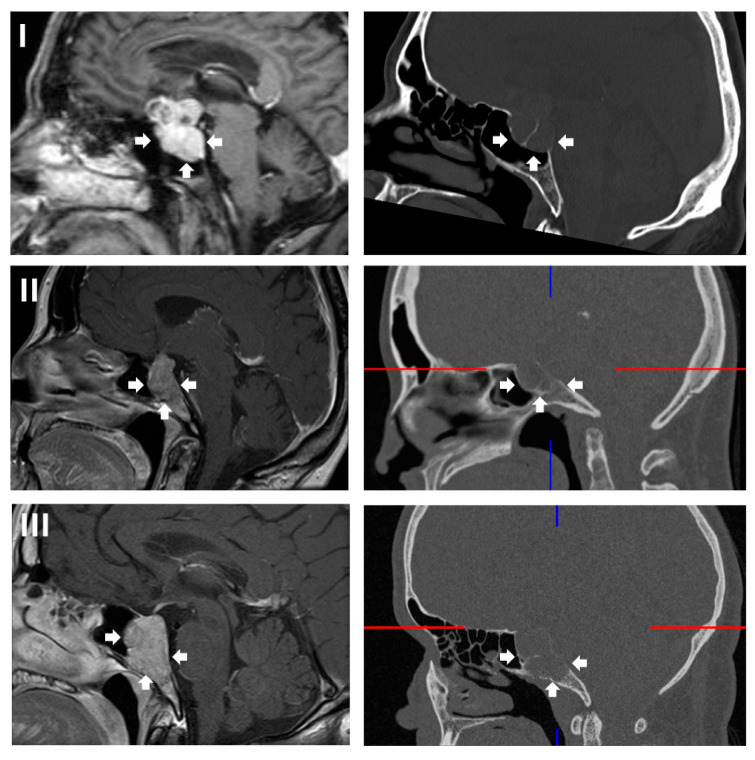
Examples of lesions within the respective clival regions as described in Figure 2. Left: contrast enhanced, T1-weighted cranial magnetic resonance imaging; right: bone window cranial computerized tomography. (I) The lesion is predominantly within the sella; the sellar bone and dorsum sellae are thinned diffusely; the tumor also presents smaller suprasellar extensions. Region II is spared by the tumor. (II) The lesion grows almost entirely in a downward direction within regions I and II. The sellar bone is largely intact, while the cortical lamella to the clivus is breached focally, enabling invasion of the cancellous clivus. The cortical lamella to the posterior fossa remains intact. (III) The tumor grows exclusively downwards and within all three clival regions. The anterior wall of the bony sella and floor of the sphenoid remain intact, while the sella floor is focally perforated, enabling tumor invasion of the cancellous clivus. A thin cortical lamella to the posterior fossa remains. The tumor extension is highlighted by white arrows. The color lines are an artefact of three dimensional image reconstruction.
